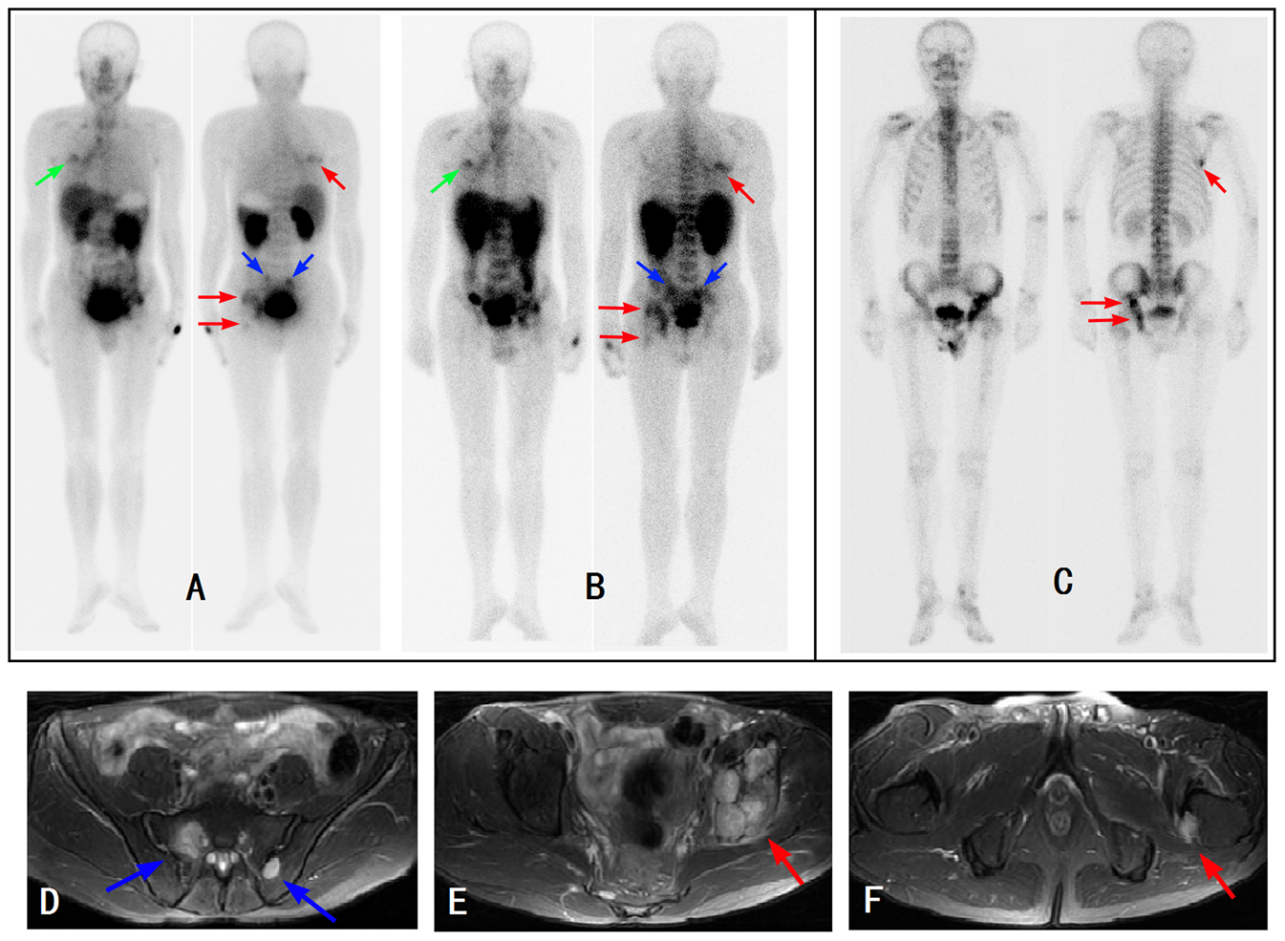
A 48 year-old man with right lung adenocarcinoma (green arrow) and multiple bone metastases (red and blue arrows). ^99m^Tc-3PRGD2 scans showed two more bone metastases (blue arrows) than the ^99m^Tc-MDP bone scan. The lesions were confirmed by MRI (lower row). A: 1-h ^99m^Tc-3PRGD2, B: 4-h ^99m^Tc-3PRGD2, C:^ 99m^Tc-MDP, D–F: MRI.
